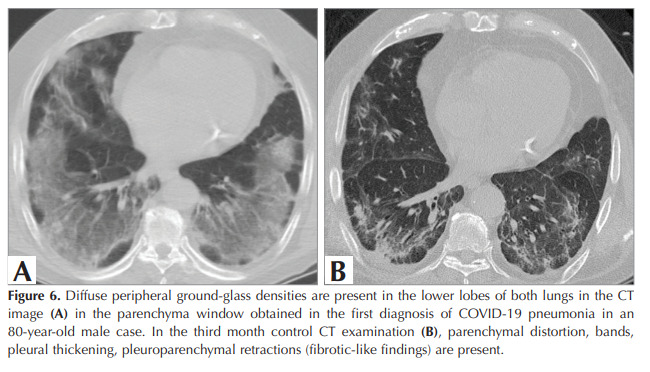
Diffuse peripheral ground-glass densities are present in the lower lobes of both lungs in the CT
